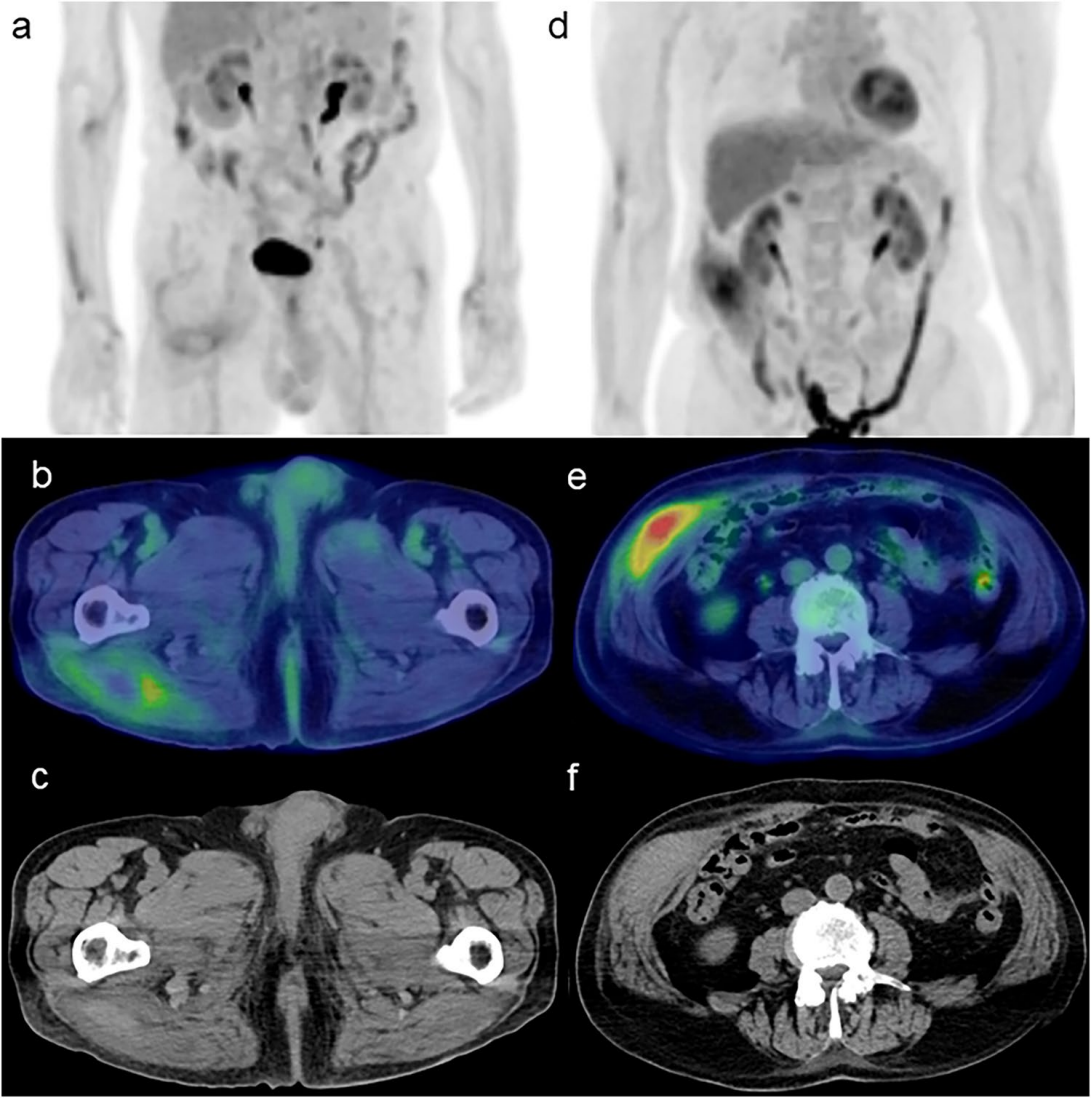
a, d FDG-PET MIP images. b, e Axial FDG-PET/CT images. c, f CT images. The region of high FDG uptake in swollen muscle corresponds to the hematoma apparent on CT as a region of relatively high attenuation
